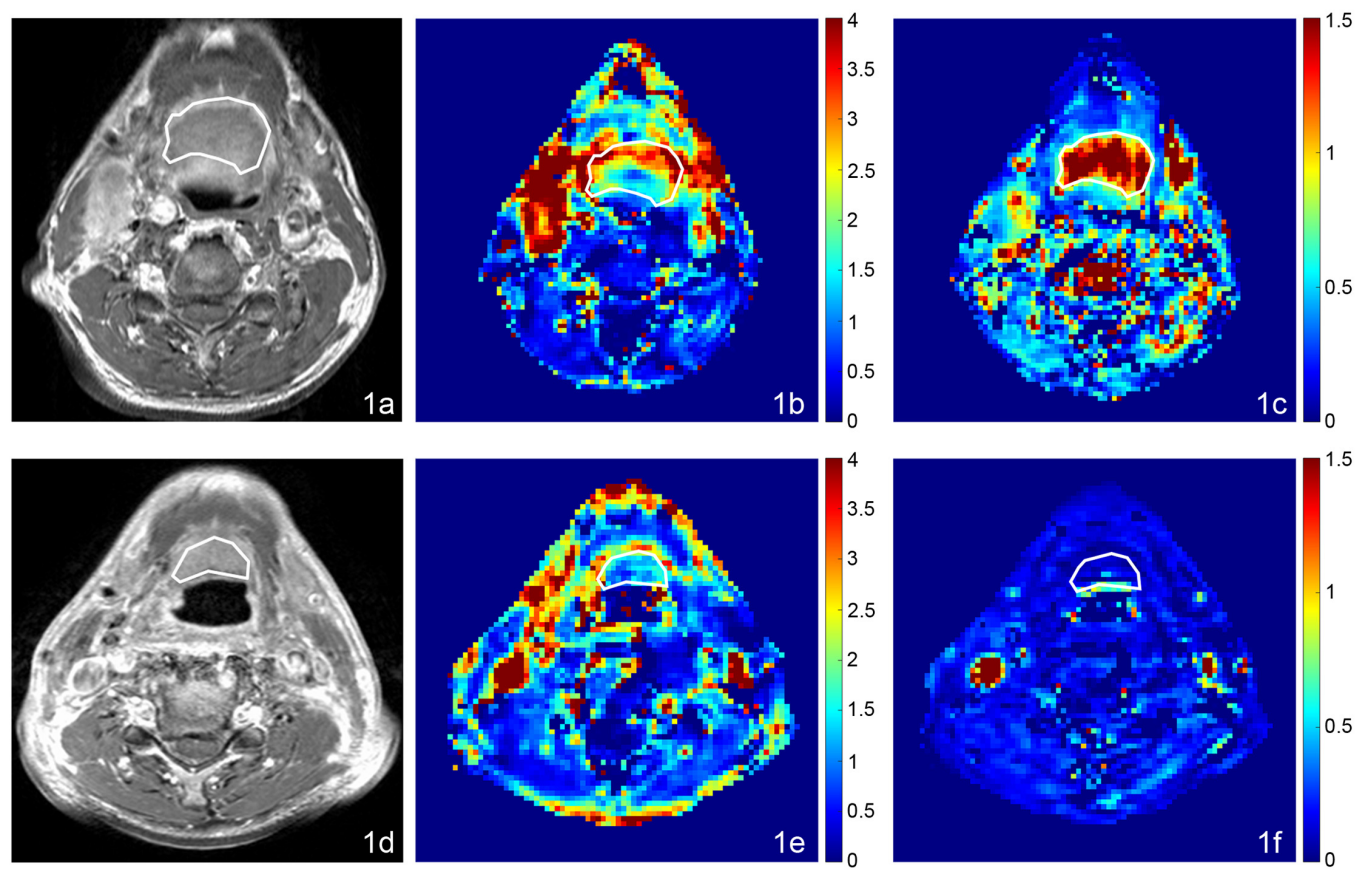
MRI, AUGC and k_ep_ maps in the axial plane from a 70 year old male with oropharyngeal carcinoma and SC 46.7 months after chemoradiotherapy. (a) Pre-treatment T1-weighted post contrast image of the SCC, (b) Pre-treatment AUGC map (mean AUGC = 2.36), (c) Pre-treatment k_ep_ map (mean k_ep_ = 1.62); (d) Post-treatment T1-weighted post contrast image of the SC residual mass, (e) Post-treatment AUGC map (mean AUGC = 0.96), (f) Post-treatment k_ep_ map (mean k_ep_ = 0.09).
